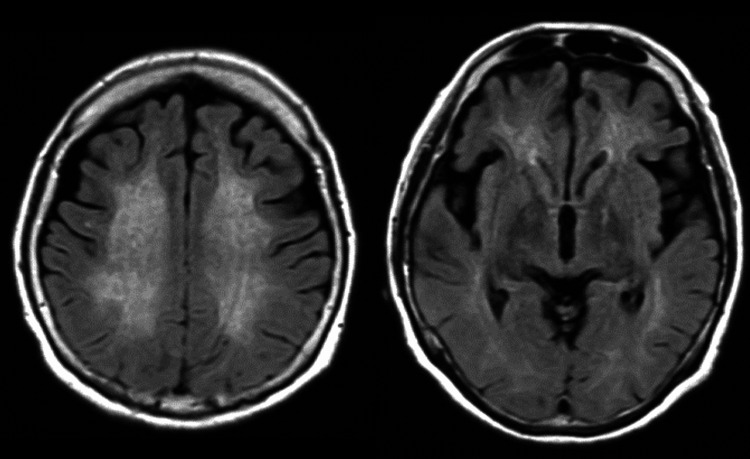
The result of T2-FLAIR brain MRI two months after onset. T2-FLAIR brain MRI showing bilateral, symmetrical hyperintensities in the deep white matter. These lesions were not noted by MRI one month after onset. FLAIR, fluid-attenuated inversion recovery; MRI, magnetic resonance imaging
